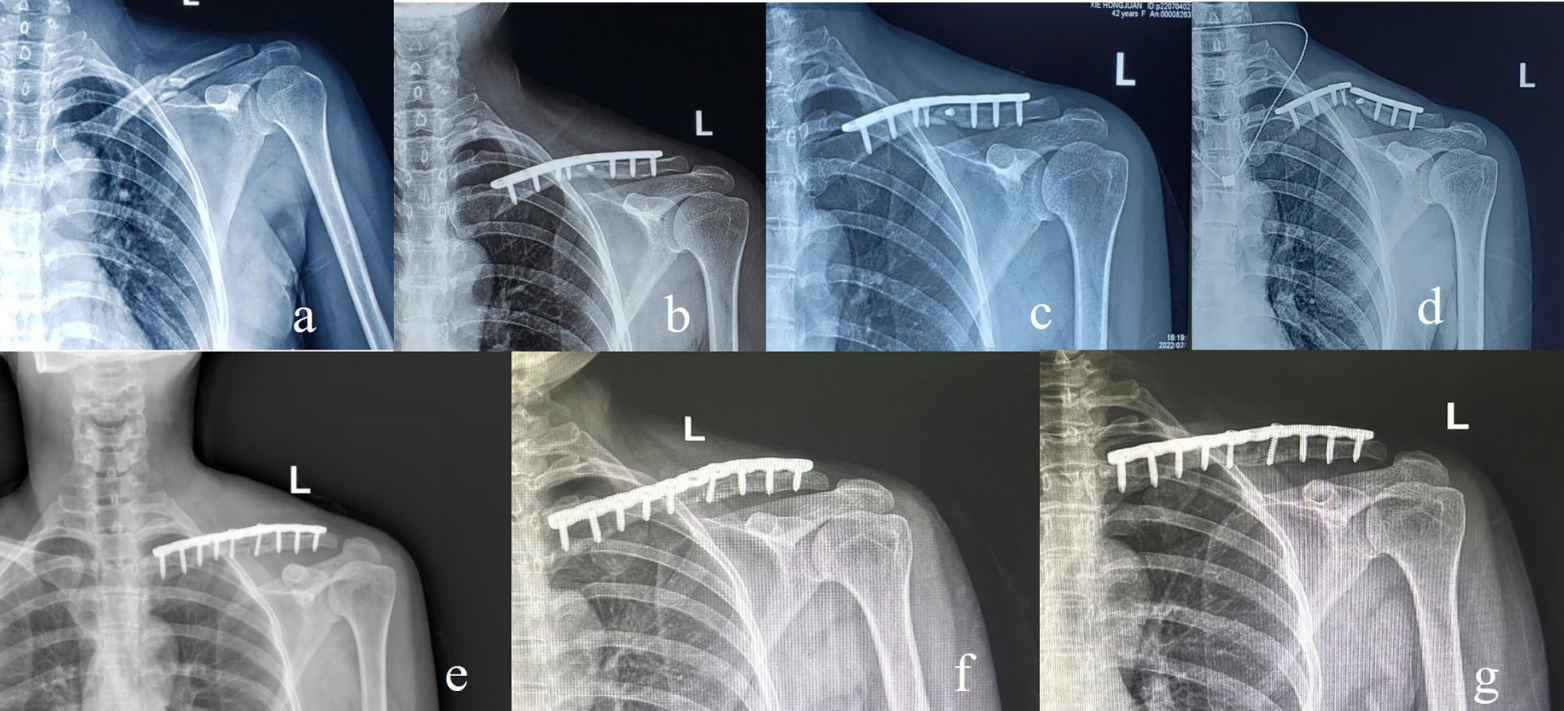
Using the ACSBG technique, a 48-year-old woman sustained a clavicular midshaft fracture as a result of a traffic accident 25 months prior. a Primitive, b postoperative, c and at 5 months postoperatively. d Plain radiographic image showing clavicular midshaft bone nonunion and plate breakage, e day 1 post-revision surgery, f the blurry fracture line, and g osseous healing of the fracture site at 3 months post-revision. ACSBG, autologous cancellous structured bone grafting
